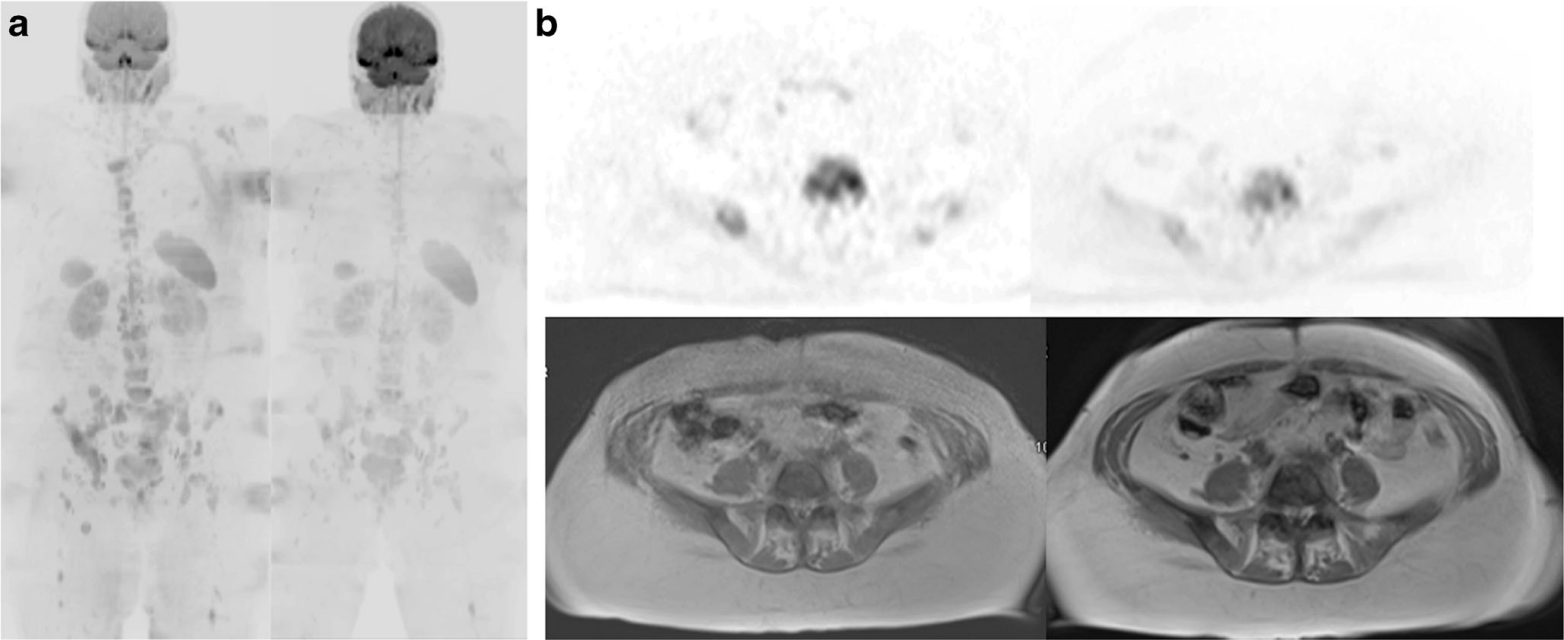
a b900 DWI MIP and b corresponding transaxial b900 DWI (*top*) and T1-W (*bottom*) images at baseline (*left*) and 8 weeks (*right*) of a L5 lesion in the same patient as Figs. 2 and 3. ADC_med_ of the L5 vertebral lesion increased from 1047 to 1150 mm^2^/s
